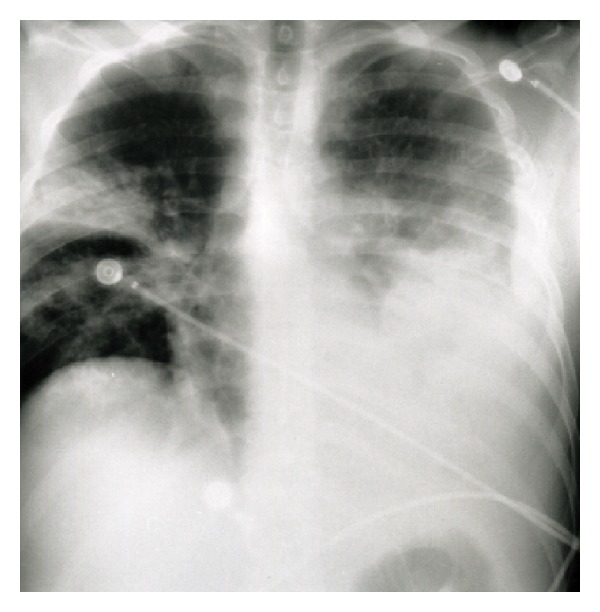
Chest X-ray on the 5th day.
